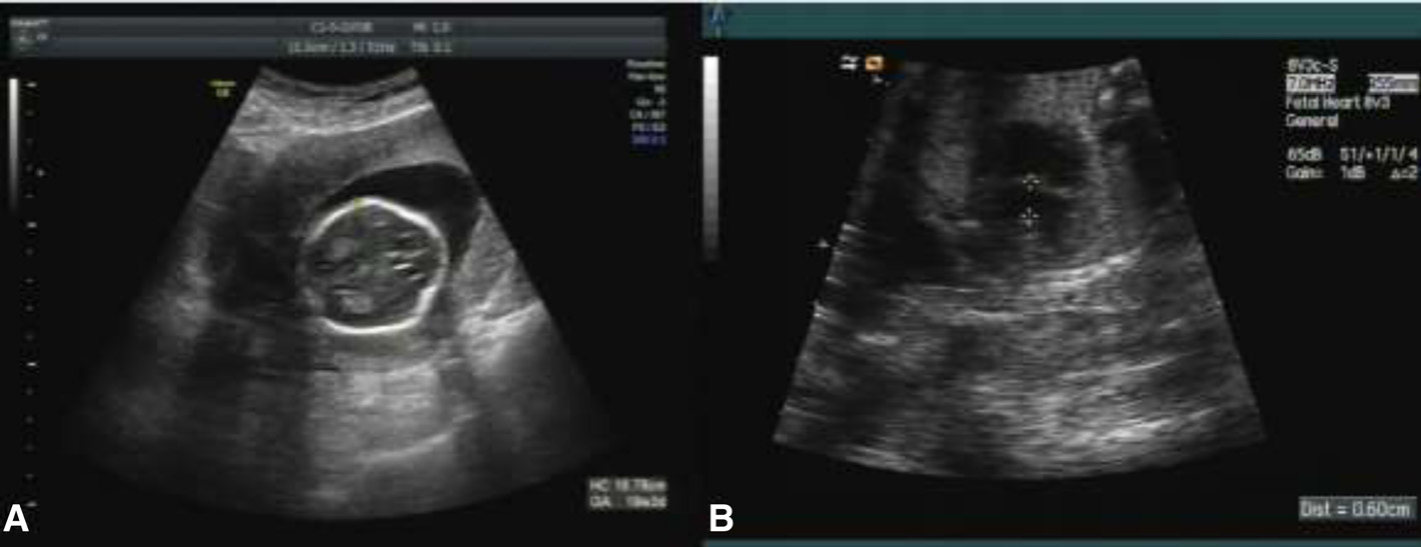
Abnormal sonographic features of fetus at 19th week. A. strawberry-shaped head. B. Four-chamber view of the fetal heart showing a ventricular septal defect (0.6 cm diameter).
